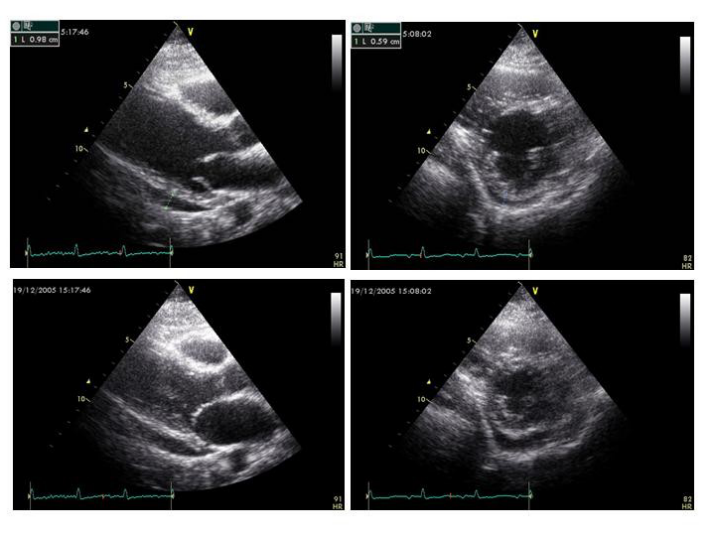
Detection of posterior pericardial effusion in a 28-year old female patient affected by non Hodgkin's lymphoma and treated by CHOP (Cyclophosphamide 750 mg/m 2 + Adriamycin 50 mg/m 2 + Vincristine 2 mg + Prednisone 100 mg) and previous mediastinal radiation therapy. The usual grading of pericardial effusion takes into account the diastolic separation between epicardium and pericardium: 1. small = < 10 mm corresponding to 300 ml, 2. moderate = 10–20 mm corresponding to 500 ml, 3. severe = > 20 mm corresponding to > 700 ml). In this patient the diastolic separation is < 10 mm (both in long and in short-axis vies), indicating mild pericardial effusion. Upper panel; visualization of diastolic pericardial effusion in parasternal long-axis (left panel) and short-axis view (right panel). Lower panel: visualization of systolic pericardial effusion in parasternal long-axis (left panel) and short-axis view (right panel).
